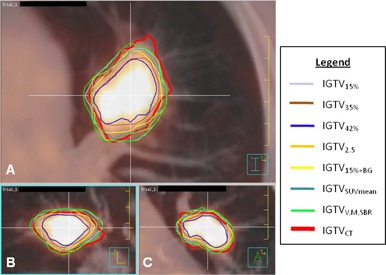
PET/CT images of tumor 1 with internal target volume (ITV) contours: (a) transverse, (b) sagittal, and (c) coronal. ${\text{ITV}}_{\text{CT}}$, shown in red, was delineated on the maximum intensity projection. Other ITVs were delineated on PET using methods described in Table 1. The VMSBR model (${\text{ITV}}_{\text{VMSBR}}$) is shown in green.
